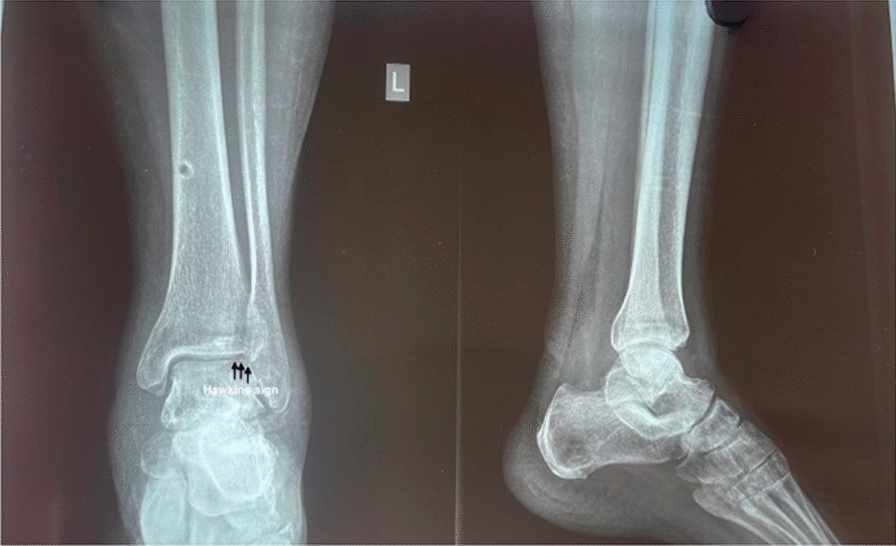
Radiograph 6 months post injury
